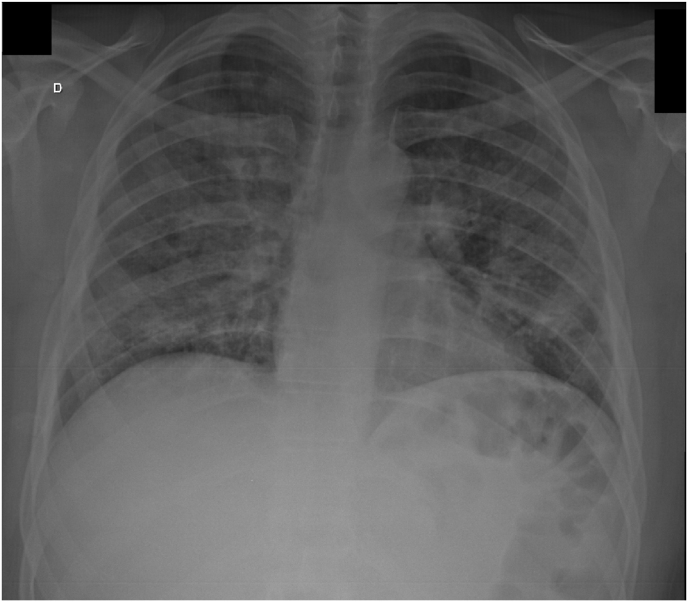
Chest X-ray with diffuse bilateral reticular infiltrate.
